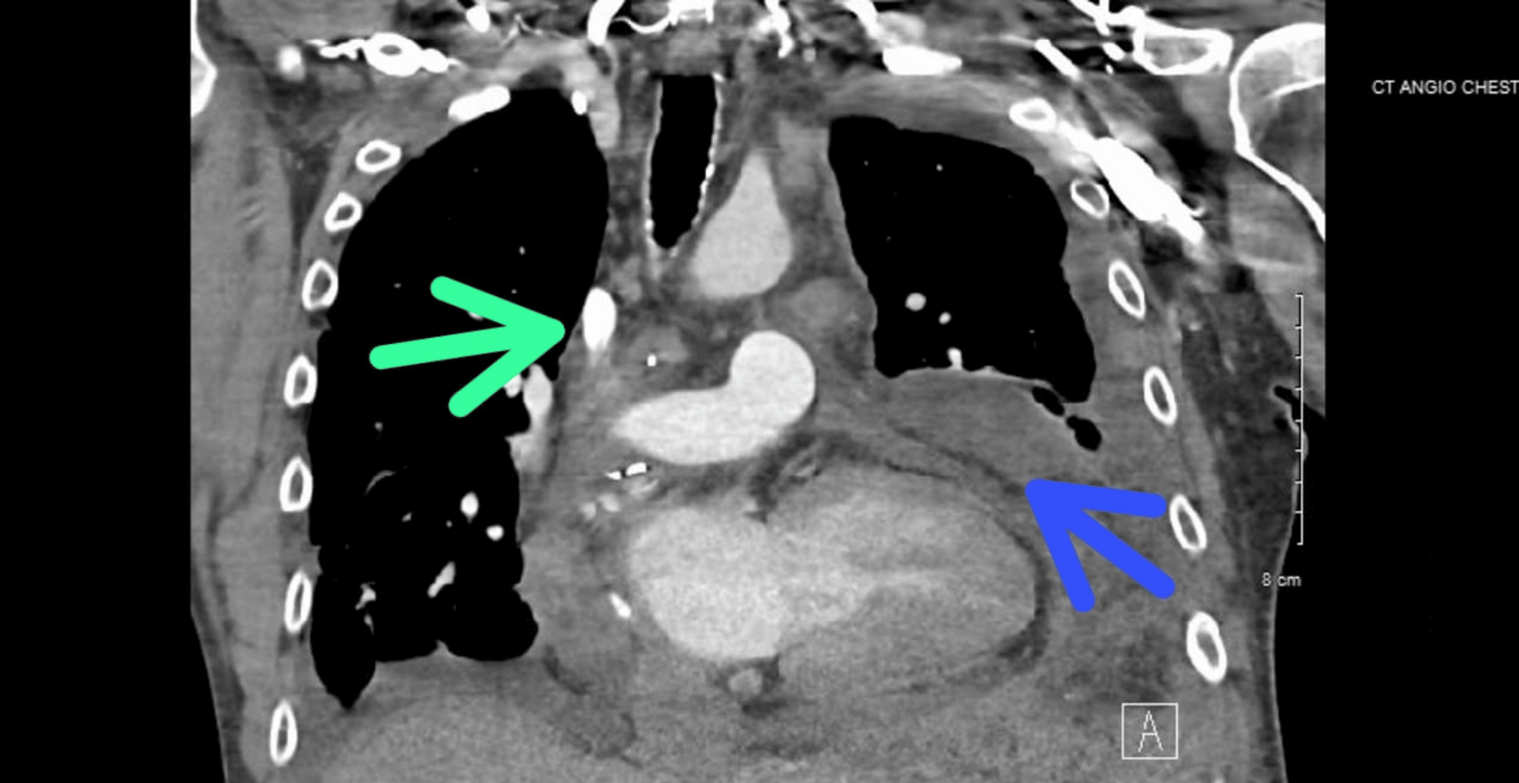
Coronal view of CT angiogram of the chest showing mediastinal lymphadenopathy (green arrow) and left subpleural opacity (blue arrow) CT: computed tomography
